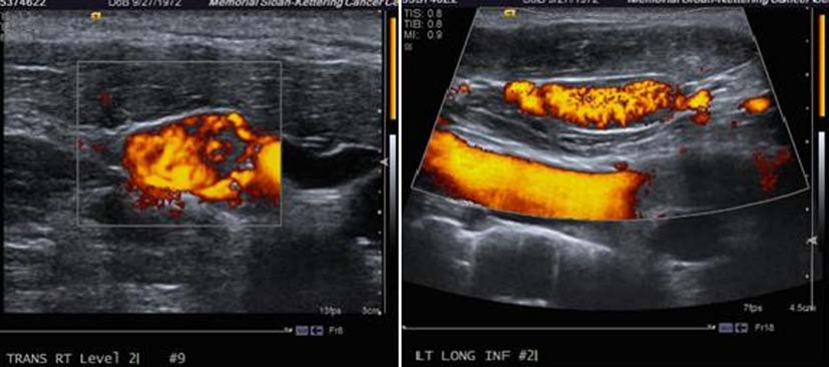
Ultrasound of the neck showing a thyroid mass suspicious of malignancy.
